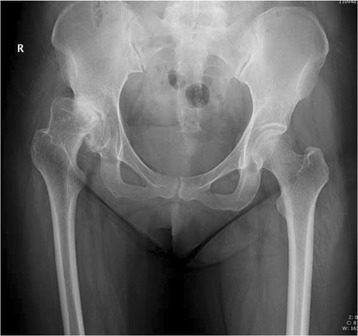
Pre-operative radiographic aspect in an anteroposterior view of a 40-year-old female with Crowe grade II hip dysplasia on her right side. The patient presented with a narrow femoral canal
